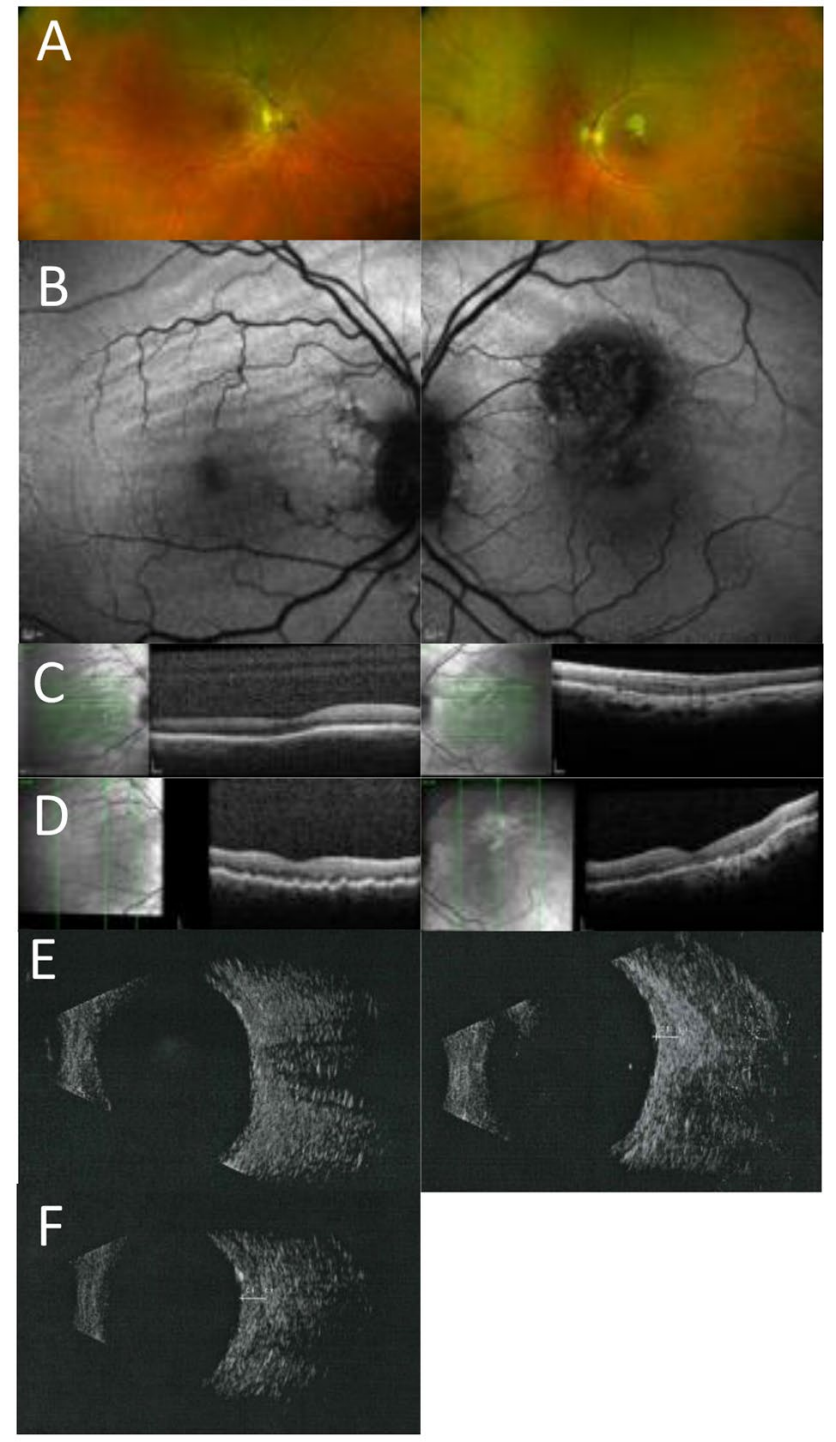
Multi-modal imaging for case 5. (A) Optos imaging showing bilateral CRF in the macular region, and in the left macula chorioretinal scarring and a supero-temporal choroidal naevus in the right eye (B) FAF imaging showing features of chronic CSC in the left eye with a reduced AF signal in the area of the chorioretinal scarring and features consistent with previous episodes of CSC in the right eye and the presence of bilateral CRF. (C-D) OCT imaging at baseline bilateral CRFs that are more obvious on the vertical scans (E) Ultrasound B scan showing bilateral flattened posterior poles and the T-Sign in the left eye (F) Thickened ocular coats and T sign in the right eye
